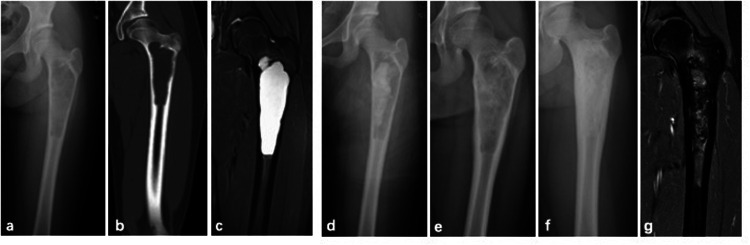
A 11-year-old female with SBC of left femur treated by PAIB. (a) Radiograph of preoperative x-ray. (b,c) CT and MRI scan. (d) Immediate postoperative radiograph. (e) 3-month postoperative radiograph. (f) 12-month postoperative radiograph showed SBC is healed. (g) MRI scan after operation 12 months.
